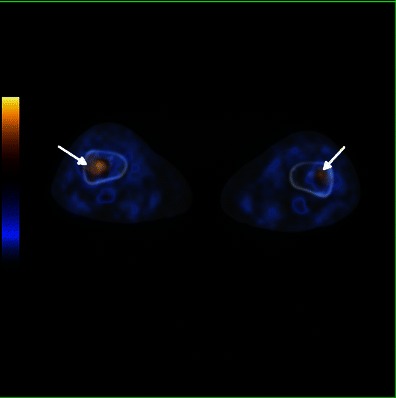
Axial FDG-PET/CT fusion images in a 71-year-old female showing elevated metabolic activity and medullary sclerosis in the long bones of the distal lower limbs (arrows)
